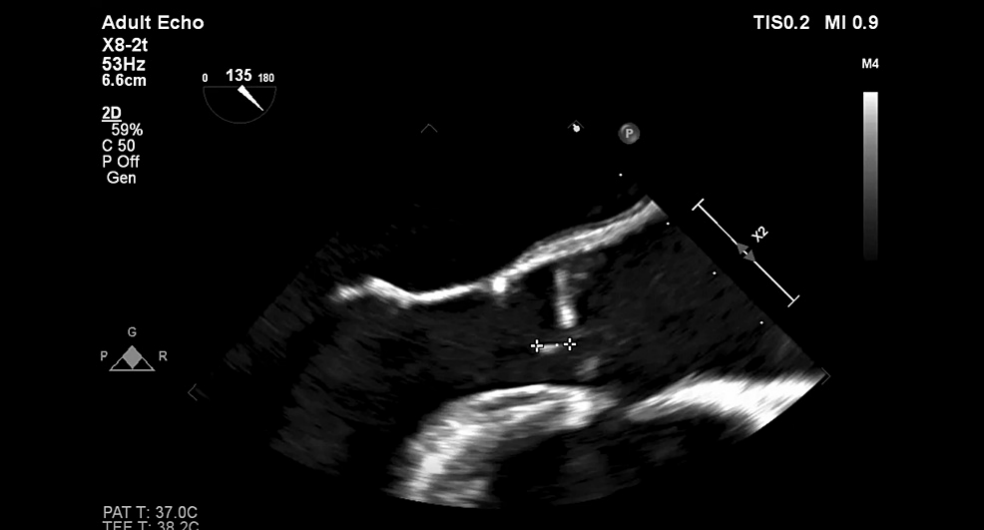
Image from echocardiogram, with evidence of endocarditis of the aortic valve.
